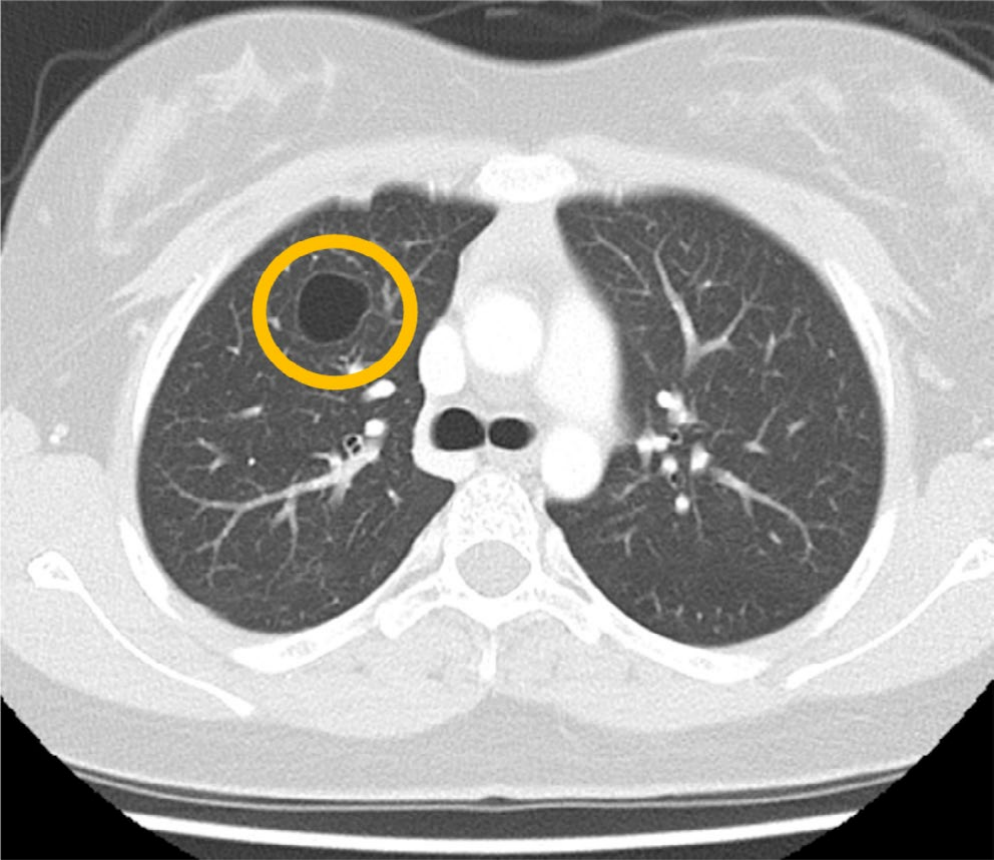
Chest CT reveals a pulmonary cyst in the right upper lobe (circle).
